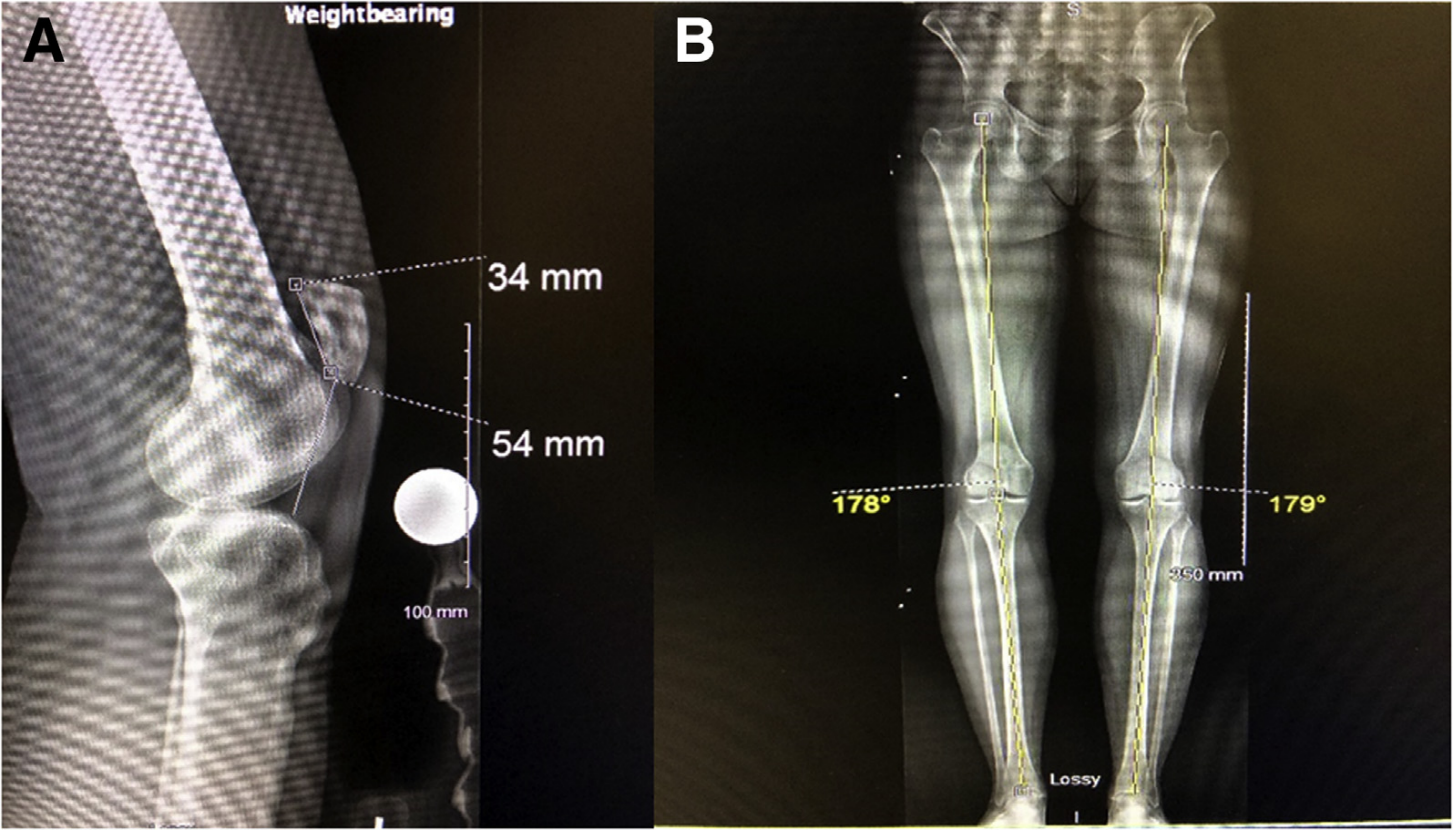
Preoperative radiographs prior to left knee tibial tubercle osteotomy. (A) A lateral radiograph shows patella alta with a Caton-Deschamps index of 1.59. (B) A standing lower-extremity alignment radiograph shows no coronal-plane malalignment.
